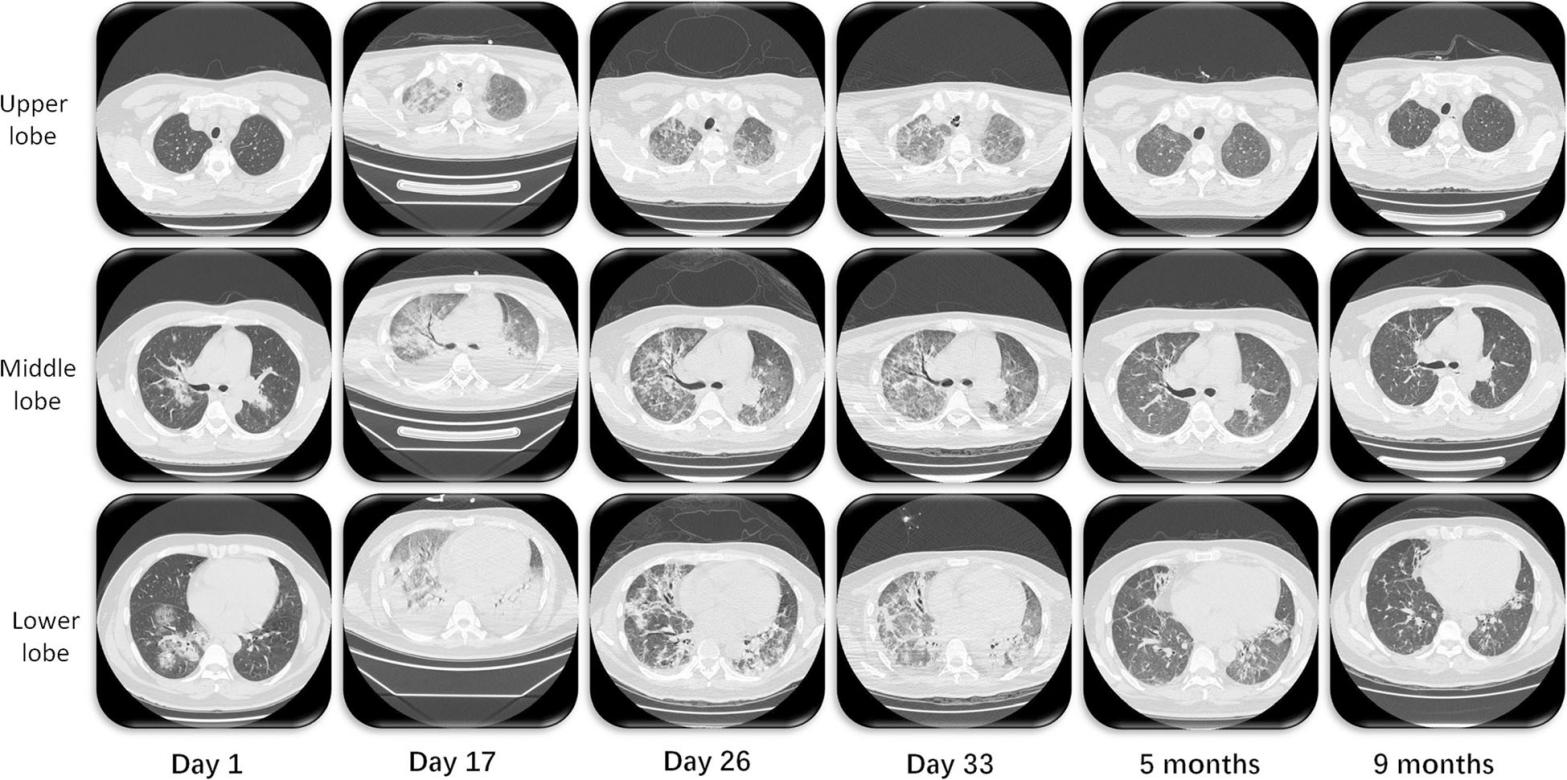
Chest computed tomography (CT) on day 1, day 17, day 26 and day 33 and followed up after 5 months and 9 months. Three representative slices of the upper, middle and lower lobe were chosen
